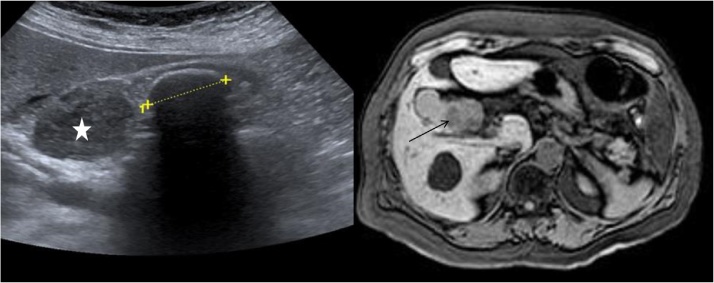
A 4,5 cm mass of the neck of the GB can be seen (marked with a star) on the left side. A gallstone is located next to the tumor. The MRI reveals a tumor marked by the black arrow.
